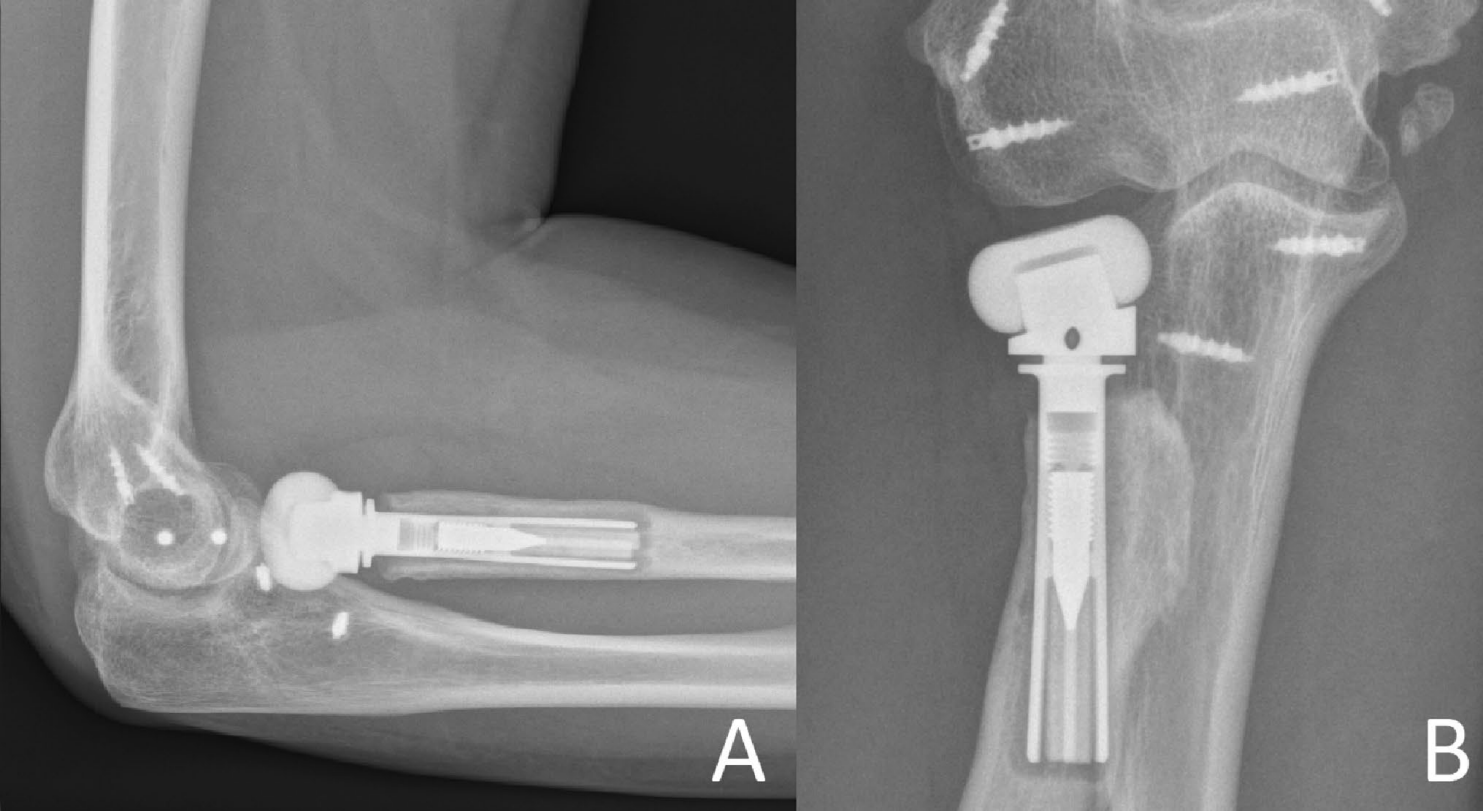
X-ray of substantial loosening of a monopolar radial head prosthesis at the shaft of the proximal radius (a lateral, b a.p)
